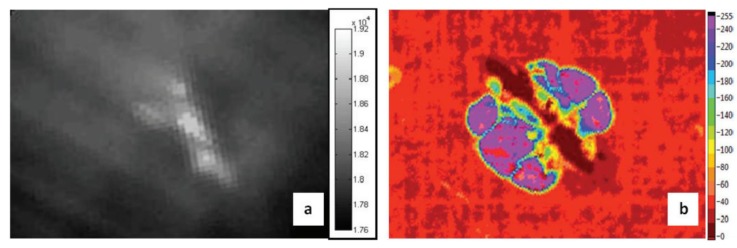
Comparison between eddy current stimulated thermography (a) and ultrasonic C-Scan (b) on impact damaged CFRP sample, with permission from [139].
